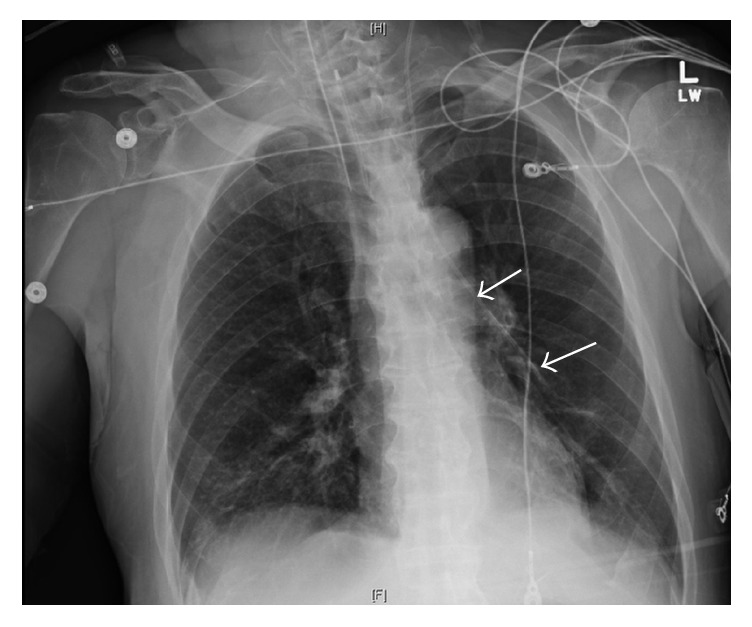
Inspiratory chest X-ray showing left internal jugular central catheter in aberrant left superior pulmonary vein (arrows).
